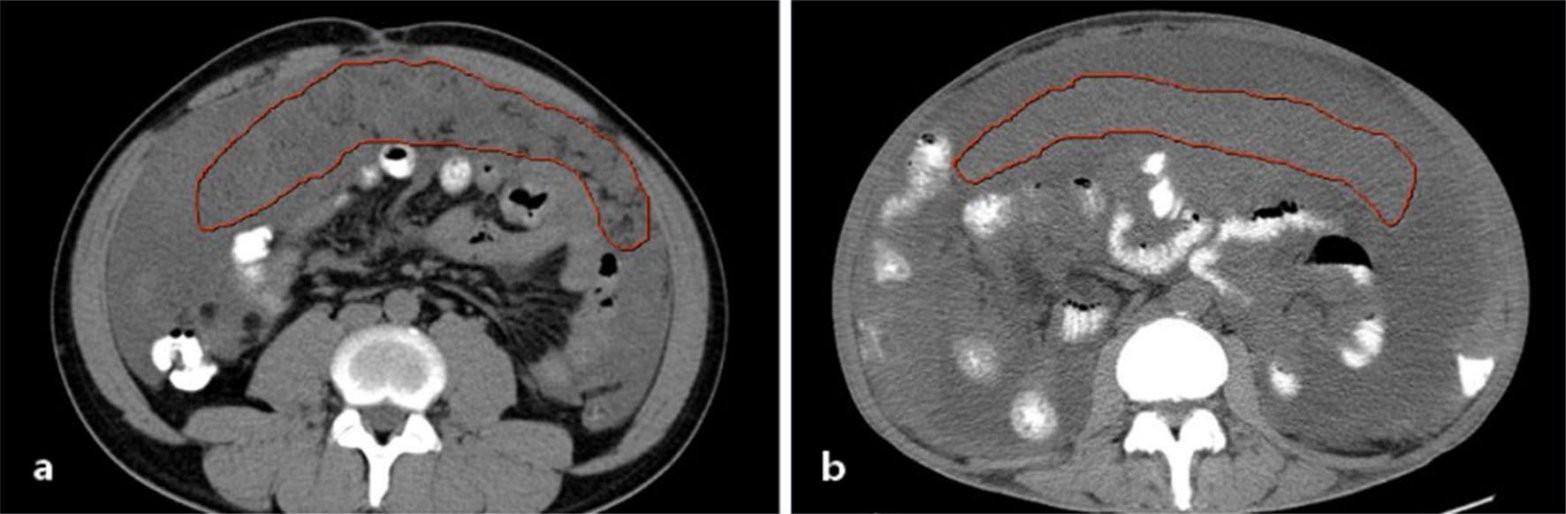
The CT ROI area of the greater-omental caking. (a) A 37-year-old man with low-grade PMP; the red curve shows greater omental caking. (b) A 62-year-old woman with high-grade PMP; the red curve shows greater omental caking.
